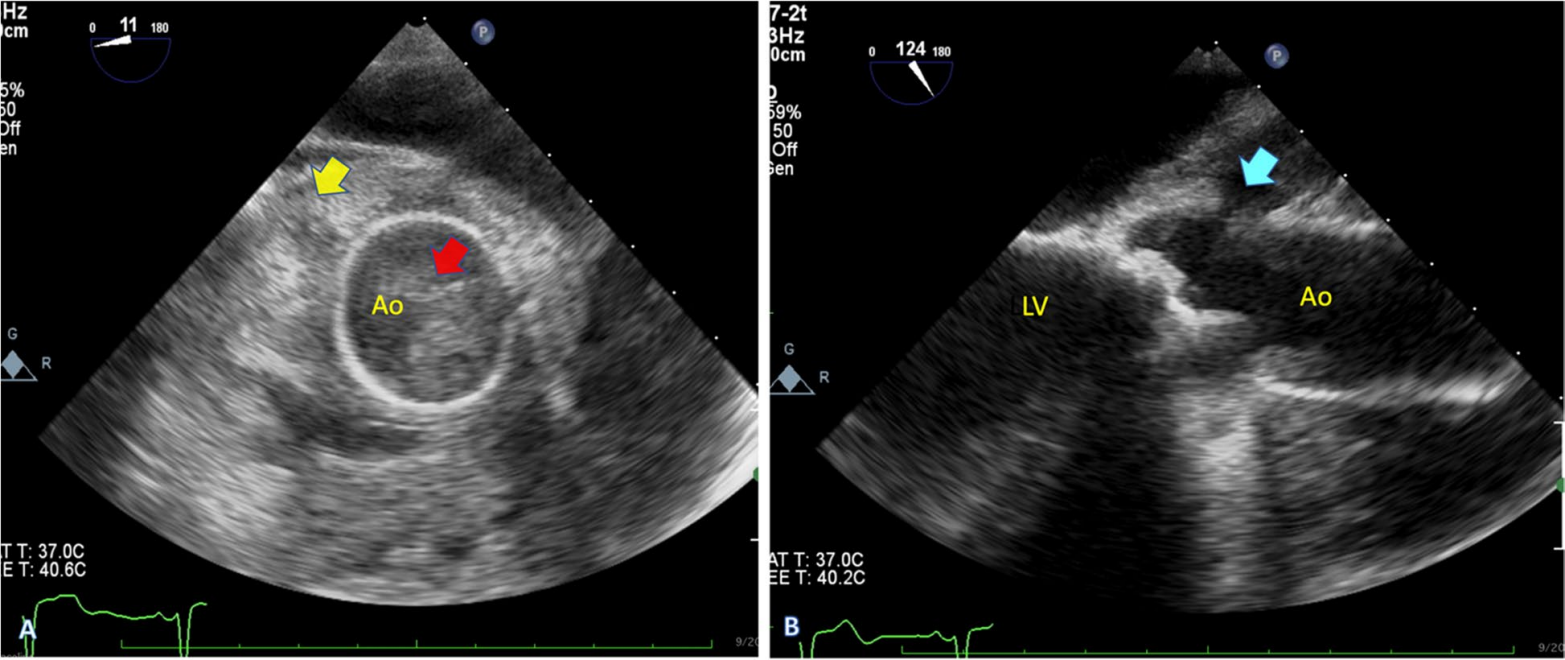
Transesophageal echocardiography: diffuse multiple masses (red arrow) in the tubular aortic graft with extension to the peri-aortic space (yellow arrow), A large disruption (cyan arrow) and pseudoaneurysm in the posterior part of the tubular graft. Ao, Ascending aorta; LV, Left ventricle
